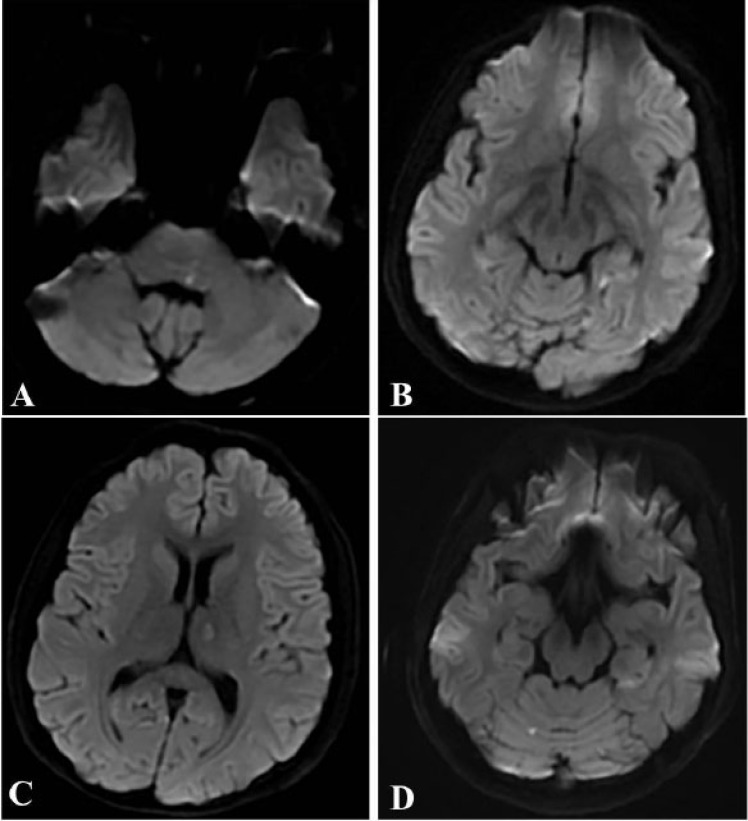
Brain MRI. Acute infarction in the (A) left pons; (B) occipital lobe; (C) thalamus; and (D) right cerebellum.
